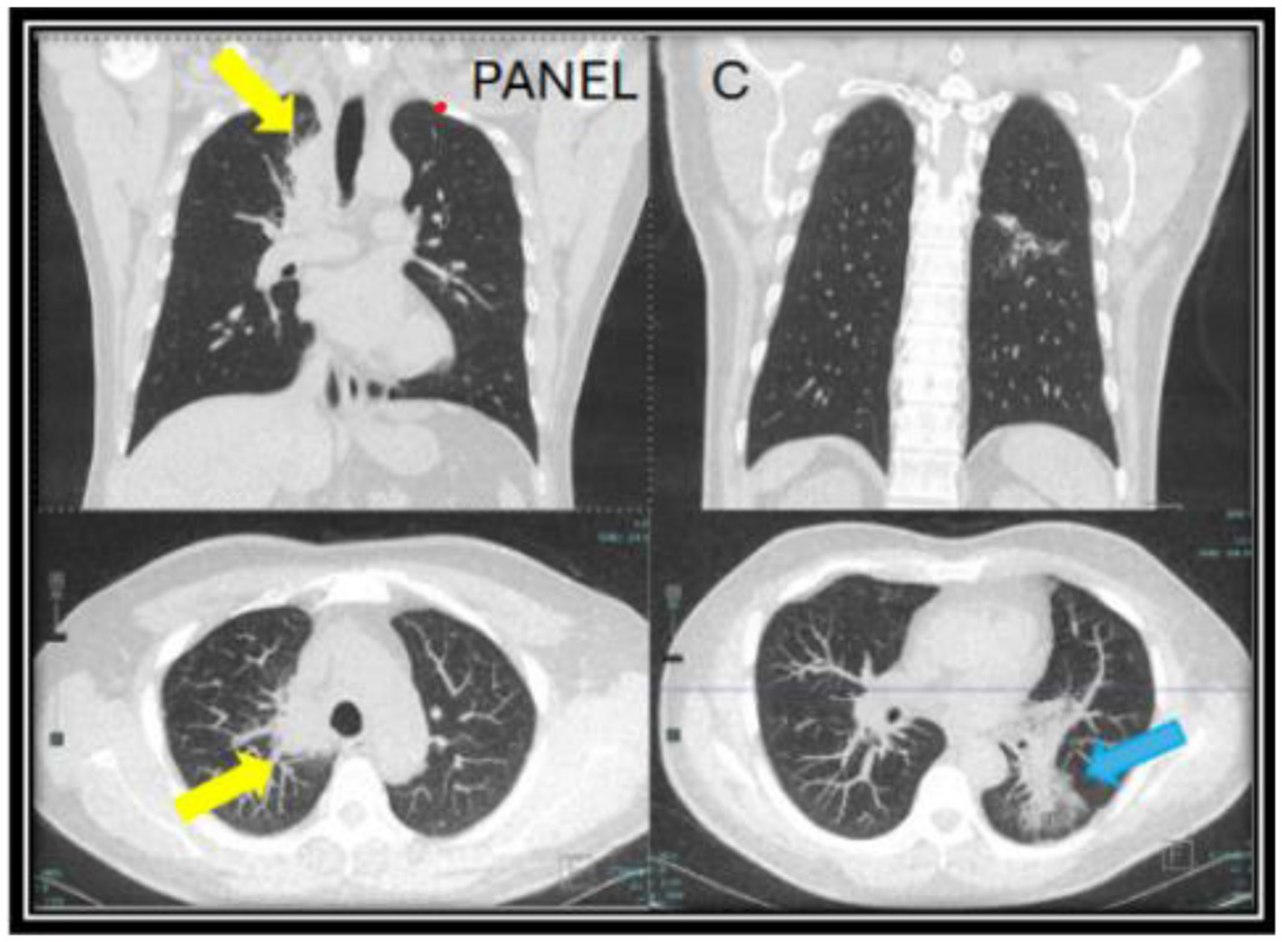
CT chest images taken a year after of being off of itraconazole (year two) when the patient developed a new persistent cough associated with a rise in Coccidioides complement fixation titers. A new mass-like consolidation of the medial right lung apex measuring 5.1 × 2.9 × 0.8 cm (yellow arrow) was noted. The superior segment of the left lower lobe continued to improve (blue arrow). The nodular consolidation has decreased, and mild scarring and bronchiectasis now persisted in this area (C).
